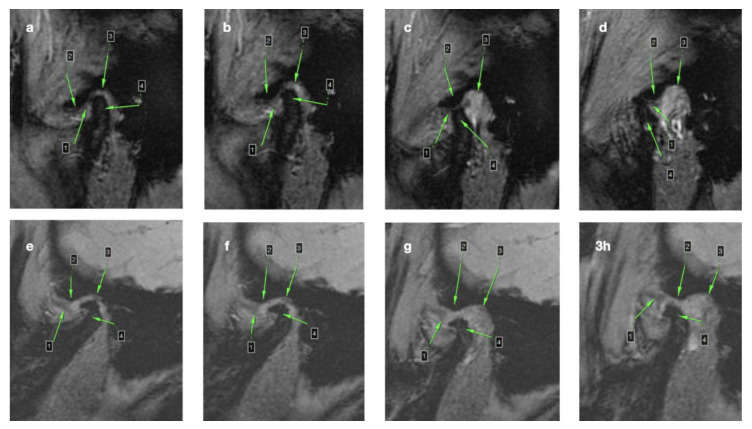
MRI images coming from dynamic sequences, presenting condyle and articular disc movements during mouth opening in two different conditions: (a–d) Normal disc position (the disc moves above the condyle forward to the top of the articular eminence); (e–h) Anterior disc displacement without reduction with osteoarthritic change-osteophyte (the disc is positioned anteriorly to the condyle; while the mouth is being opened, the articular disc does not reduce to its central position above the condyle); /1/Articular disc; /2/Articular eminence; /3/The roof of glenoid fossa; /4/Condyle.
